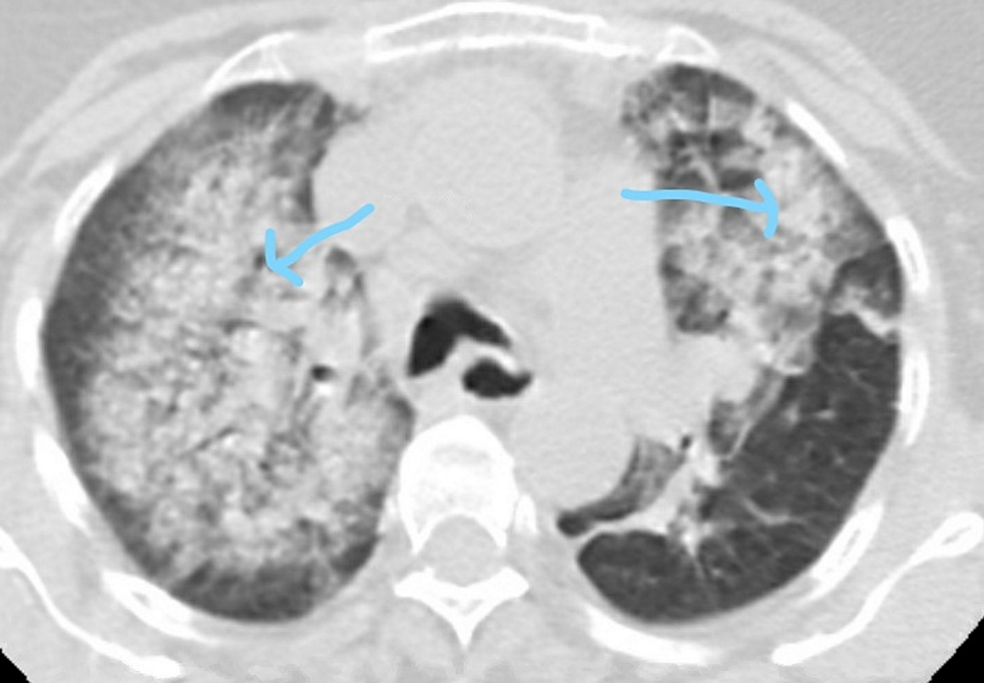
Axial Reconstruction of Chest Computed Tomography of Case 2 Arrowheads point to airspace disease involving the right and left upper lung lobes concerning for diffuse alveolar hemorrhage (DAH)
